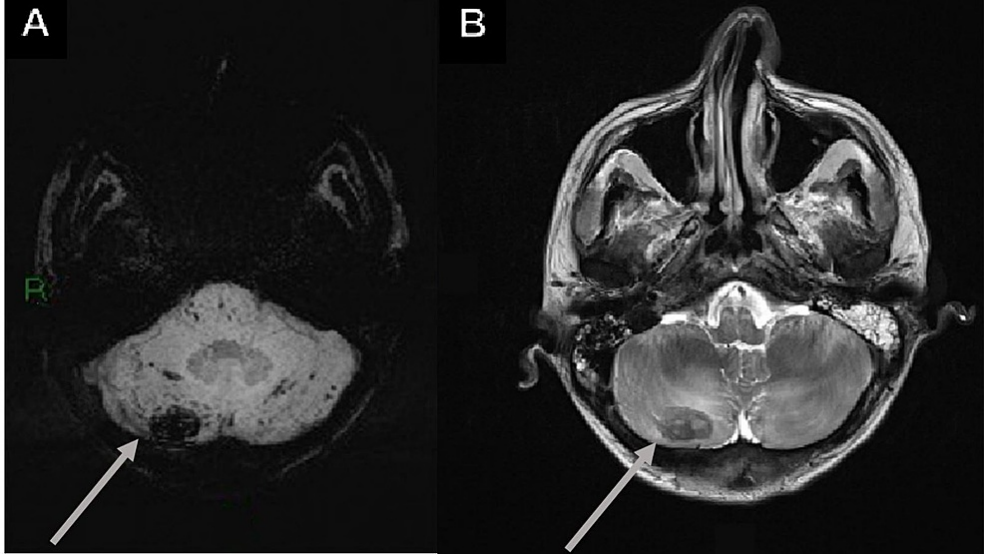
Cerebellar microbleeds and cerebellar hemorrhage in a 30-year-old patient. (A) SWI axial image with brainstem and cerebellar hemisphere microbleeds. (B) T2WI with right cerebellar hemorrhage and perilesional edema. SWI: susceptibility-weighted images; T2WI: T2-weighted image
